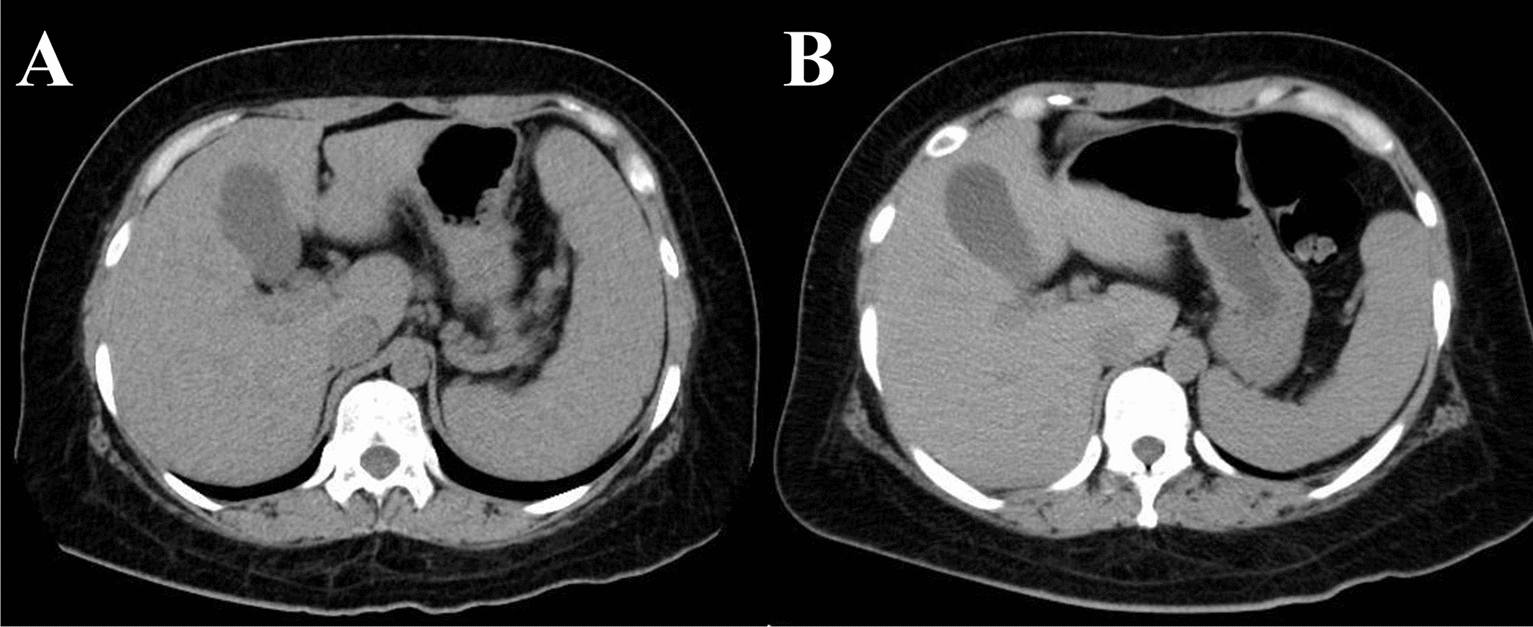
Computed tomography: A on admission to the authors’ hospital; and B 3 weeks after treatment initiation. The patient had significant splenomegaly on admission, which improved after the treatment
